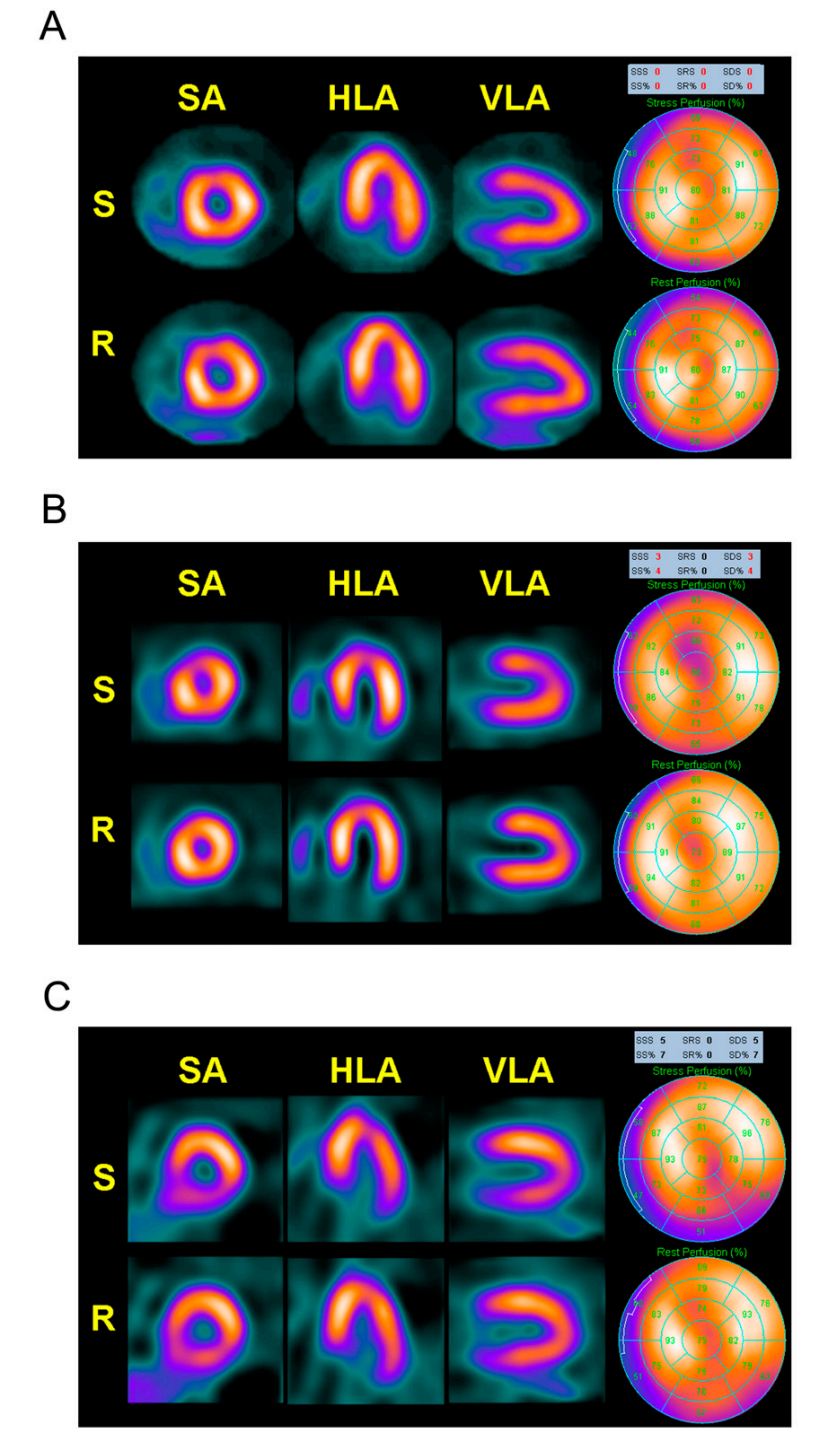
Images corresponding to myocardial perfusion SPECT obtained in diabetic and non-diabetic control subjects. A) Normal SPECT in a representative non-diabetic subject. B) Mild reversible defect in the left ventricular antero-apical region (SDS=3) in a representative type 2 diabetic patient. C) Moderate reversible defect in the left ventricular inferior region (SDS=5) in a representative type 2 diabetic patient. HLA: Horizontal Long Axis, R: Rest, S: Stress, SA: Short Axis, SDS: Summed Difference Score, SRS: Summed Rest Score, SSS: Summed Stress Score, VLA: Vertical Long Axis.
